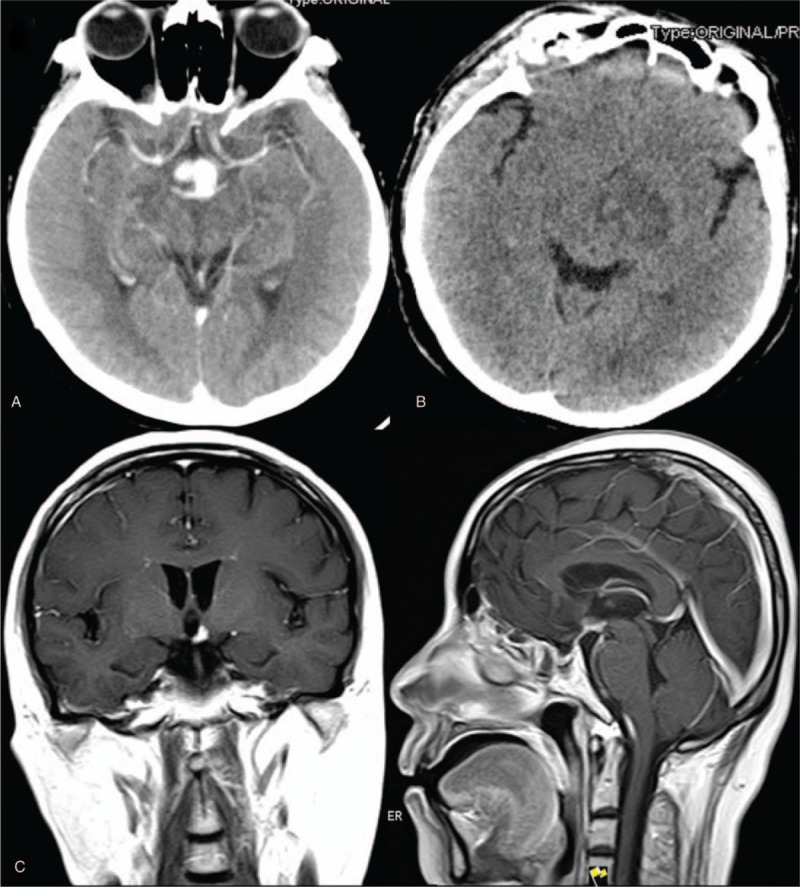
Head CT and MRI in 2015. A) Pre-operation CT showed soft tissue density shadow (1.6 × 1.3 cm) with irregular shape and well-defined margin in the sella region, and showed homogeneous enhancement. But encephalocoele, cisterns and skull bones had no abnormal signals on CT image. B) Post-operation CT showed the bone flap of the right frontal bone with scalp soft tissue swelling and accumulation of gas in surgical area. A mixture of air, edematous fluid, and hemorrhage were seen under the bone flap. Right frontal lobe was slightly compressed. There was intracranial air, thin layer of subdural edematous fluid in bilateral frontotemporal area and left side of falx cerebrum. C) MRI showed postoperative manifestation of the sella region. There were the diploe signal disorder and thickening dura around the right frontal bone. An enhanced 1 cm-diameter patch was in the fundus of the 3rd encephalocoele. Pituitary and pituitary stalk were thin.
